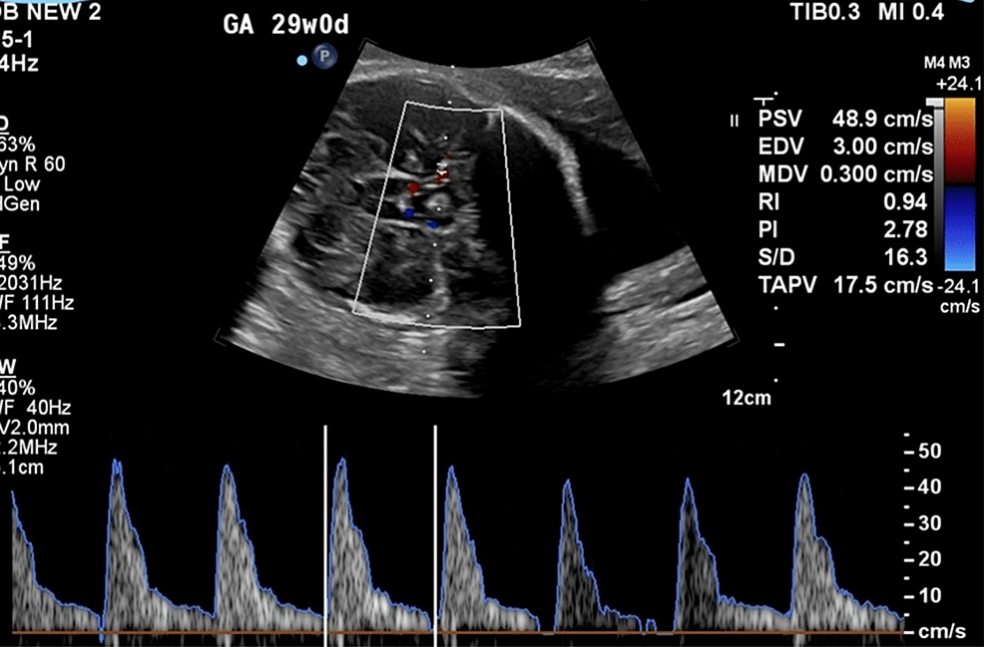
MCA Doppler Measurements MCA - middle cerebral artery, PSV - peak systolic velocity, PI - pulsatility index
